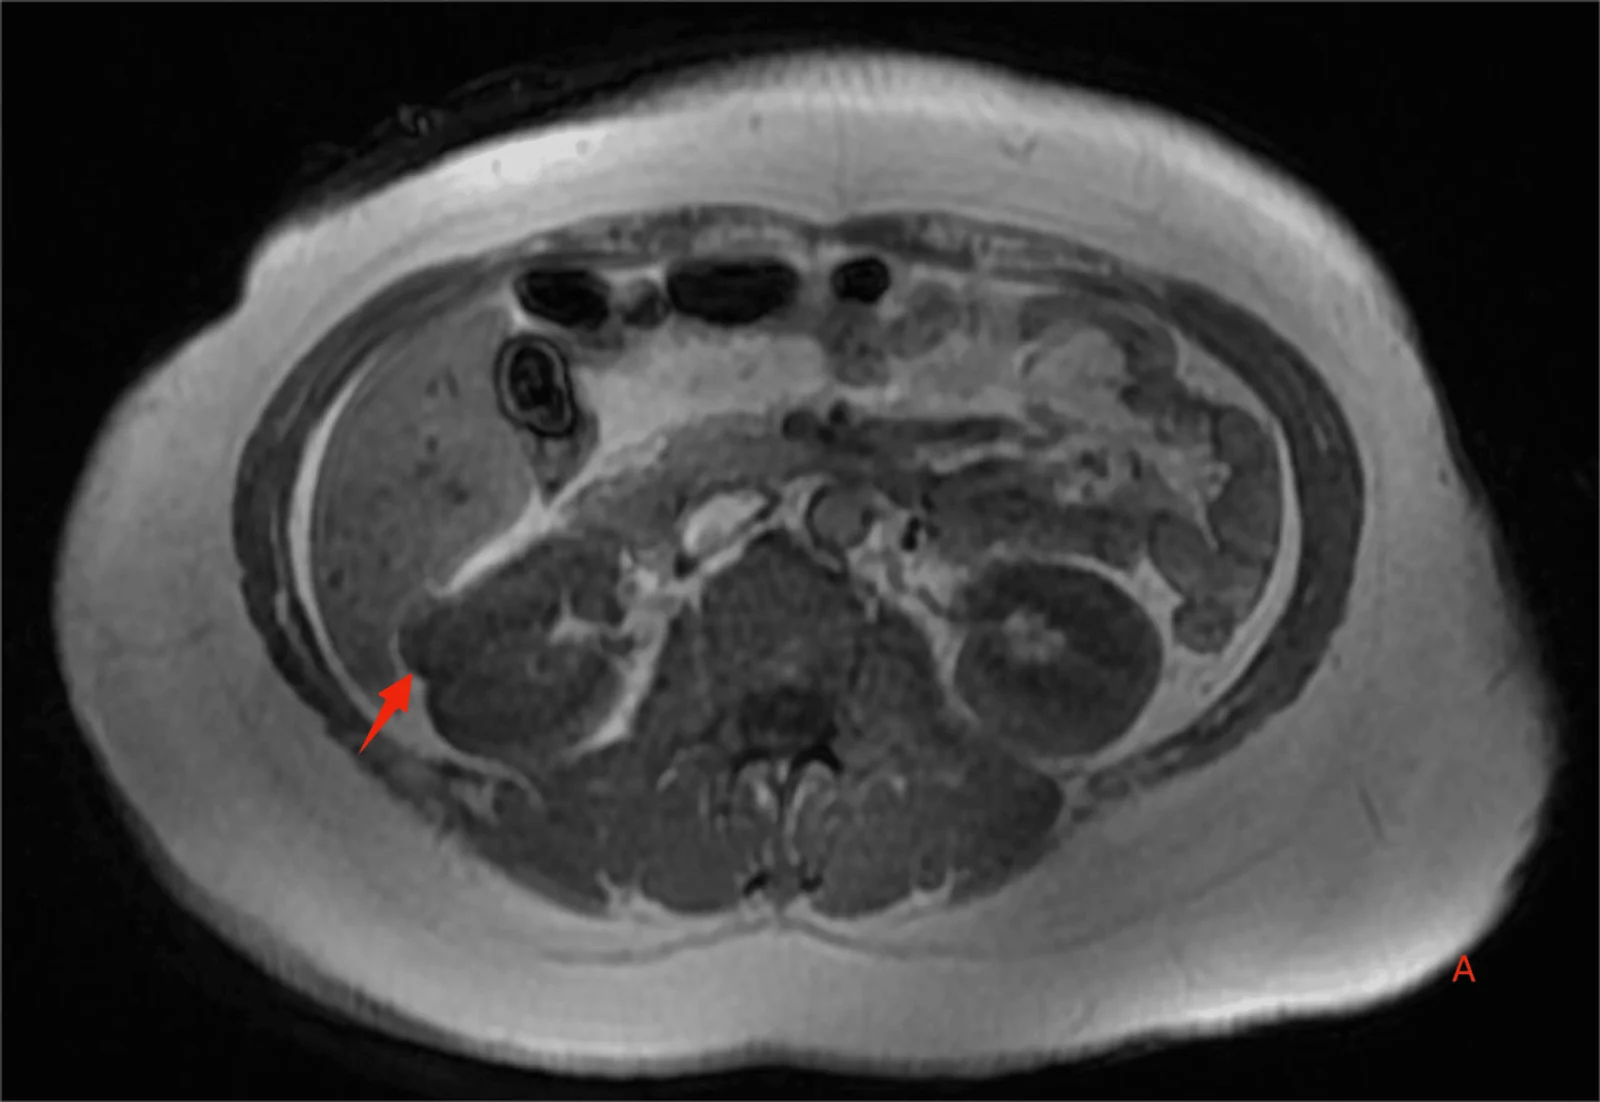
Cystic-solid lesion showing a non-uniform low signal intensity on T1WI (indicated by the arrow) T1WI: T1-weighted imaging
